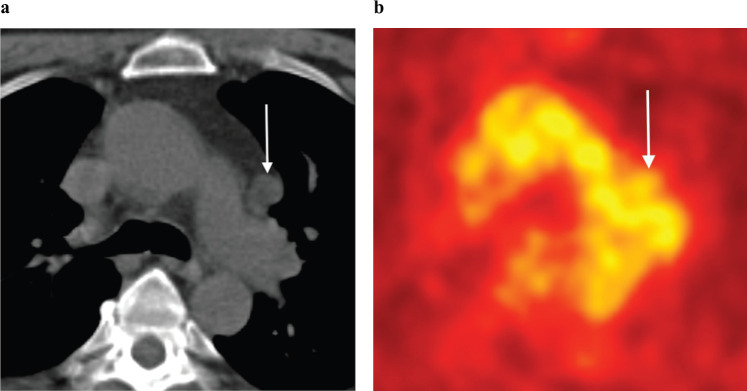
(a): Axial unenhanced CT and (b): FDG-PET images in a 63-year-old man with biochemical recurrence post-surgery and radiotherapy. There is an enlarged left aorto-pulmonary window lymph node (arrows) with no evidence of increased metabolic activity on FDG-PET. This lymph node was, however, biopsy proven to harbour metastatic prostate carcinoma. Novel PET tracers such as Choline and PSMA have greater sensitivity in detecting prostate cancer metastases.
